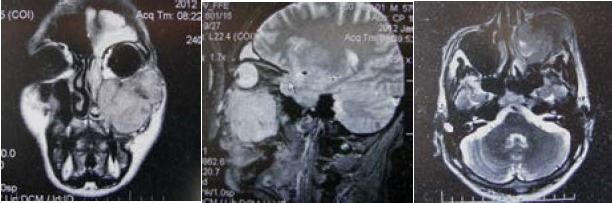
MRI of the left maxillary sinus showing that the tumor invaded the nasal cavity and the maxillary sinus
